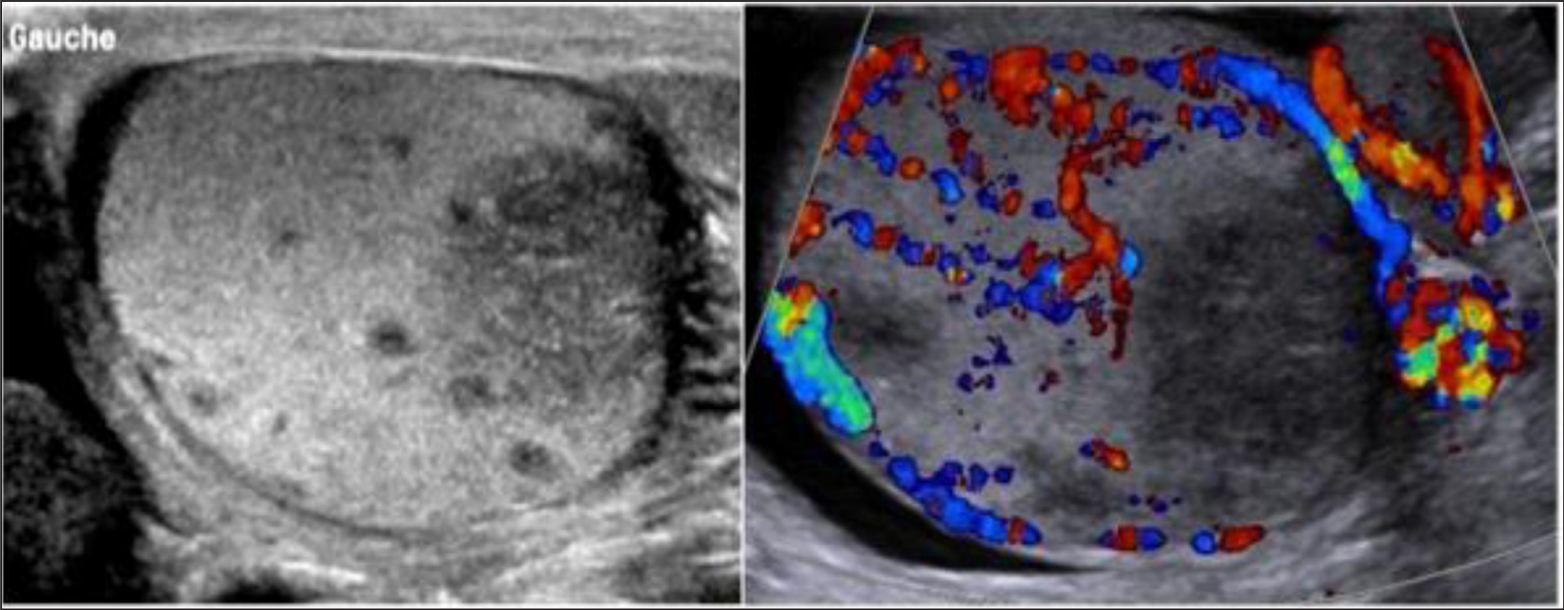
Grayscale and color Doppler of the left testis demonstrate hypoechoic avascular area of the hilum; the remaining testicular and epididymal parenchyma are more hyperechoic with flow detection.
